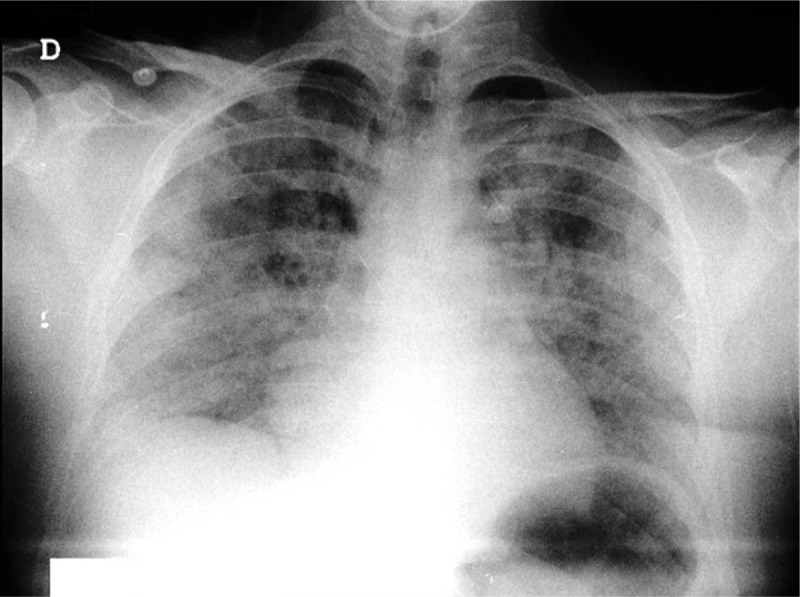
Chest radiograph upon PACU admission. PACU = postanesthesia care unit.
